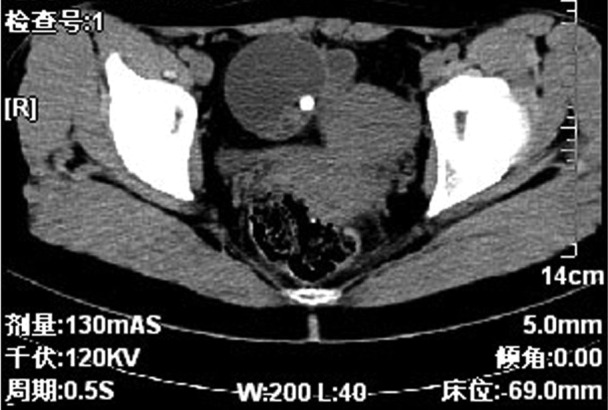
Pelvic computed tomography (CT) scan revealed a 6×4.5 cm cystic mass to the left of pelvis.
